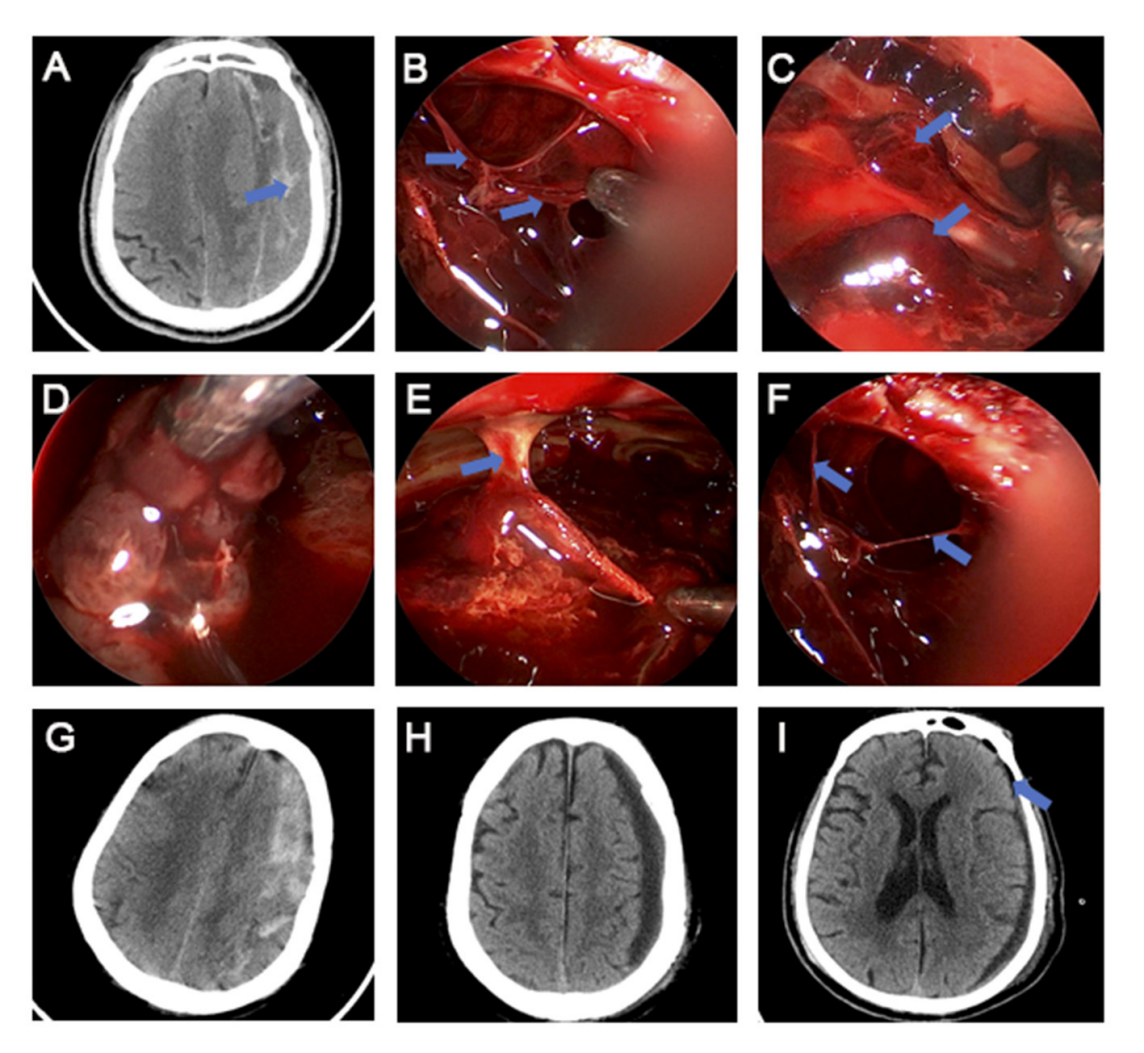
Preoperative and postoperative CT images and intraoperative images of rigid neuroendoscopy of CSDH. (A,B) Multiple-septum (blue arrow) of the hematoma. (C) Blood clots (blue arrow) that adhere closely to the capsule. (D) Suction of the incomplete liquefied hematoma. (E) A columnar trabecular structure (blue arrow) formed by the adhesion of the parietal and visceral envelope. (F) Bridging veins (blue arrow) in the hematoma cavity. (G,H) Comparison of preoperative (G) and postoperative (H) CT in a patient with the mixed density CSDH treated with rigid neuroendoscopy. (I) Little postoperative pneumocephalus (blue arrow) after rigid neuroendoscopy treatment.
